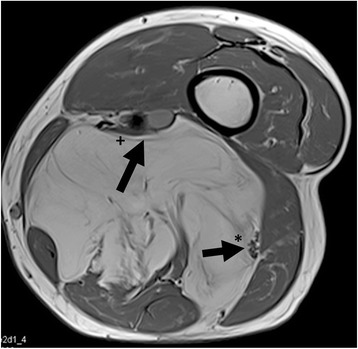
A T2-weighted MRI scan shows an atypical lipoma in the dorsal aspect of the thigh in an 81-year old patient. The sciatic nerve (*) and the major vessels (+) are close to the tumour
